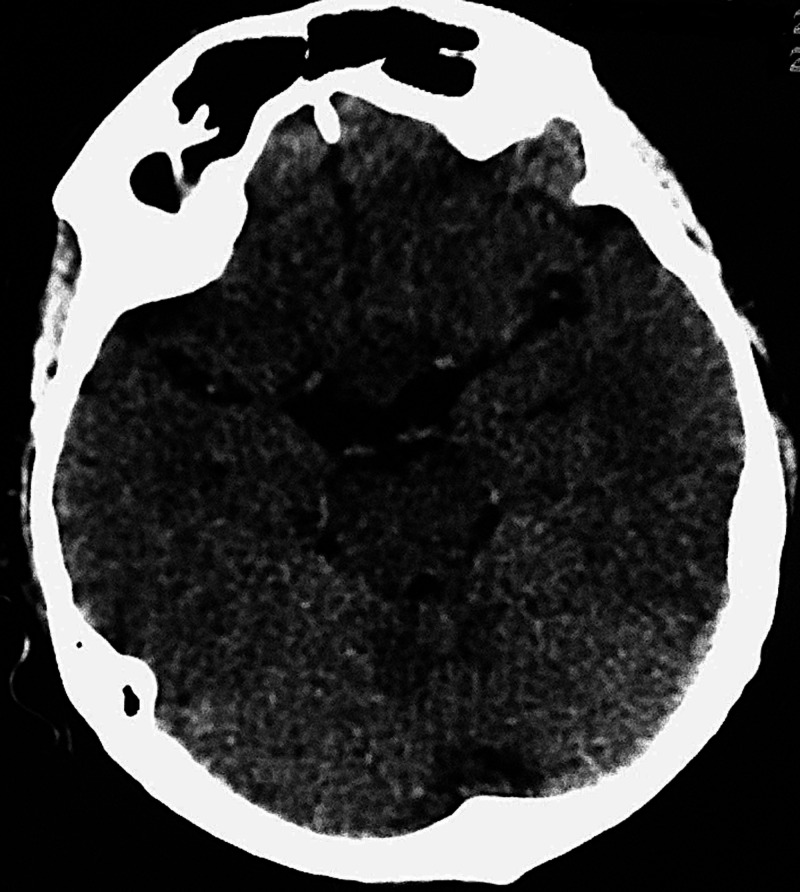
Preoperative CT CT: computed tomography
